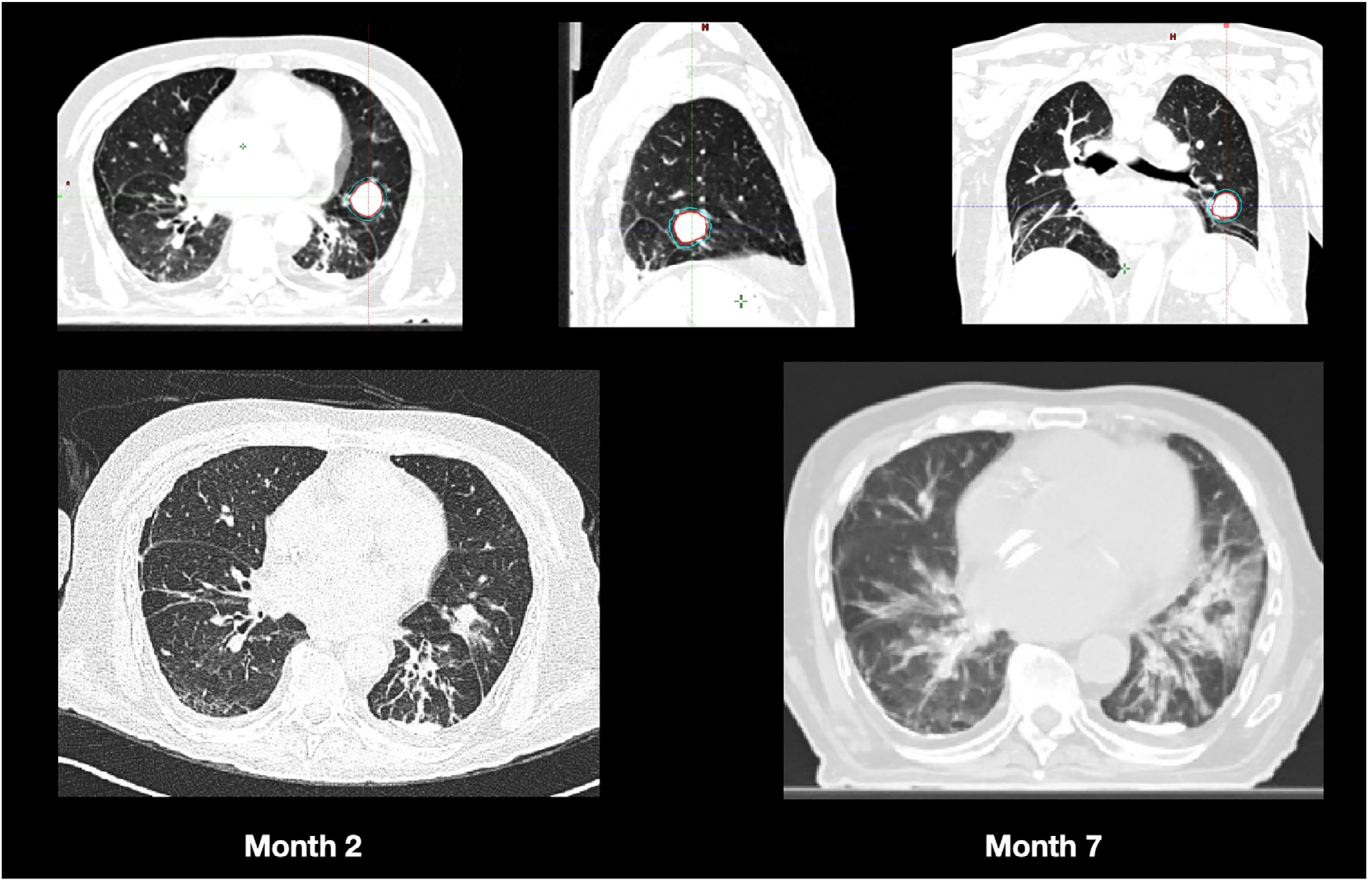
MV‐BHCT planning and follow‐up CT images of a 78‐year‐old male with metastatic colon adenocarcinoma treated for oligometastasis using the Synchrony LWR technique (GTV: 8.2 cc; PTV: 16 cc; 55 Gy/5 fractions).
